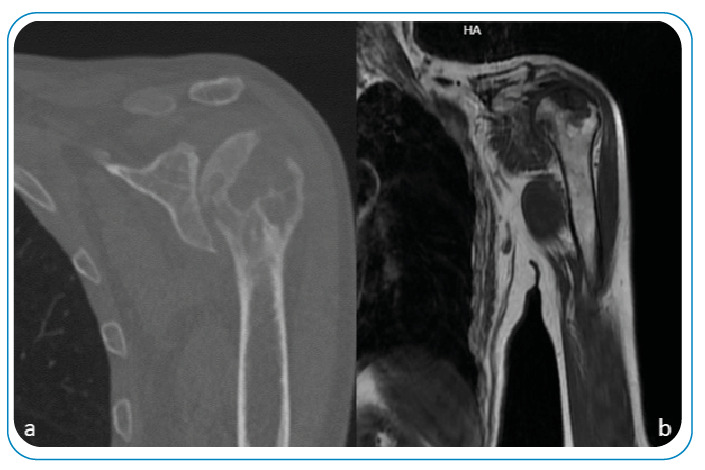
Computed tomography scan of the left shoulder showed a crescentic lucency in the humeral head (a) and magnetic resonance imaging of the patient’s left shoulder area revealed extensive hypointense erosions of the left humerus (b).
